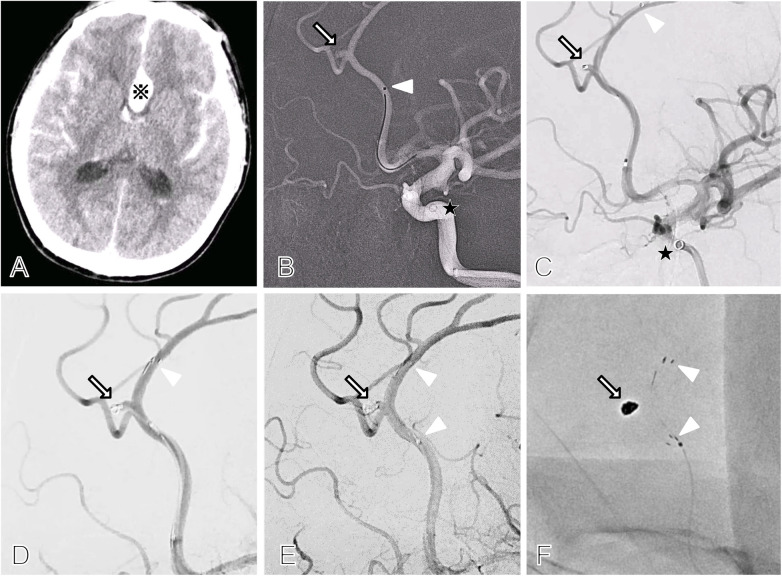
Initial imaging and Atlas^TM^ stent-assisted coiling of a ruptured distal anterior cerebral artery aneurysm. A, CT reveals extensive subarachnoid hemorrhage accompanied by an interhemispheric hematoma (※). B, Working-angle angiography shows the stent microcatheter (white arrowhead) approaching the distal anterior cerebral artery aneurysm (arrow) with support from the distal access catheter (black pentagram). C, The stent microcatheter (white arrowhead) is advanced beyond the aneurysm, while the embolization microcatheter enters the aneurysm (arrow). The distal access catheter tip (black pentagram) is positioned below the ophthalmic artery of the internal carotid artery, with the entire system having a long path and weak support. D, The distal end of the Atlas^TM^ stent (white arrowhead) is deployed. After partial stent release, the embolization microcatheter is stabilized using the jailing technique, followed by coil placement within the aneurysm (arrow). E, The proximal and distal ends of the Atlas^TM^ stent (white arrowheads) are fully deployed, achieving Raymond–Roy Occlusion Classification grade 2 coil packing with residual neck filling (arrow). The pericallosal and callosomarginal arteries remain patent. F, The mask image demonstrates the proximal and distal ends of the stent (white arrowheads) and the coil mass within the aneurysm (arrow).
